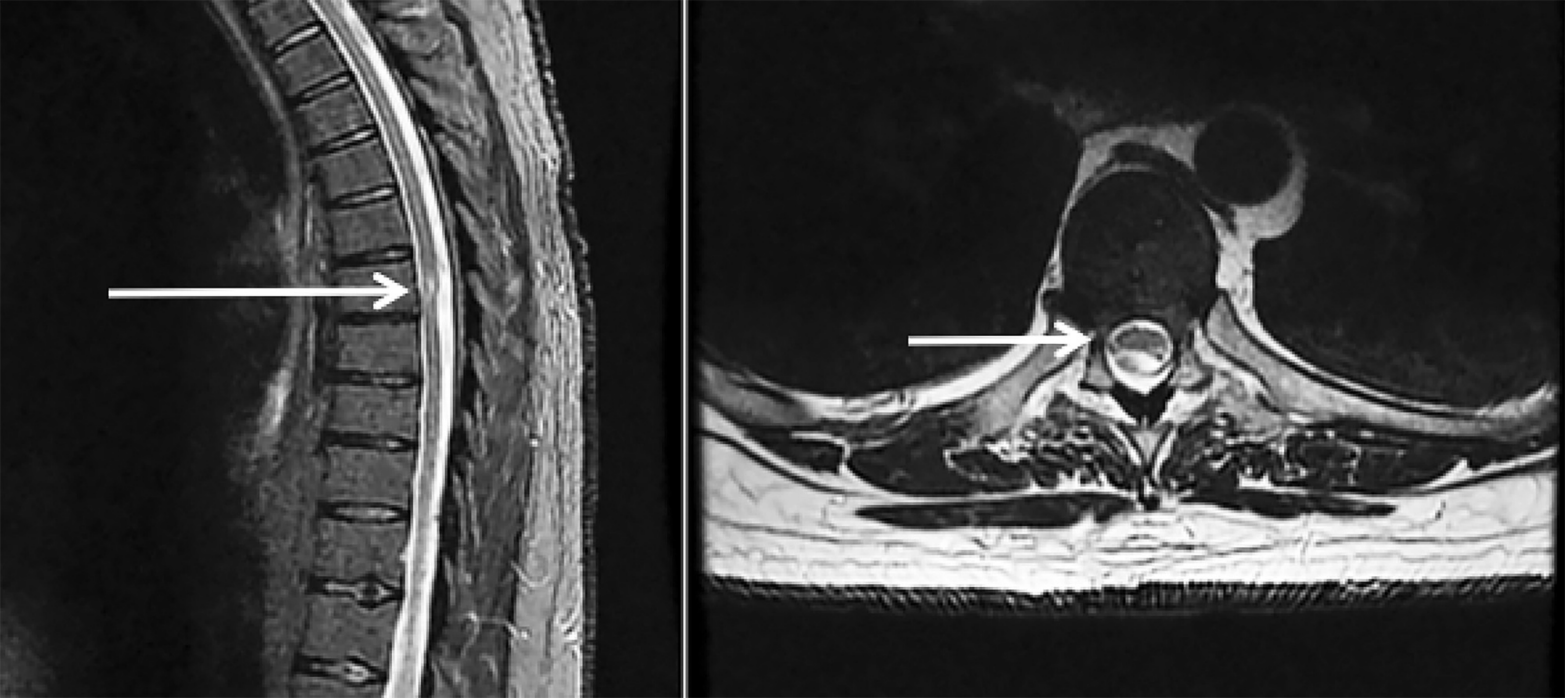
Sagittal and Axial T2 magnetic resonance imaging (MRI) demonstrating dorsal cord indentation at T7-8 concerning for possible "scalpel" sign.
